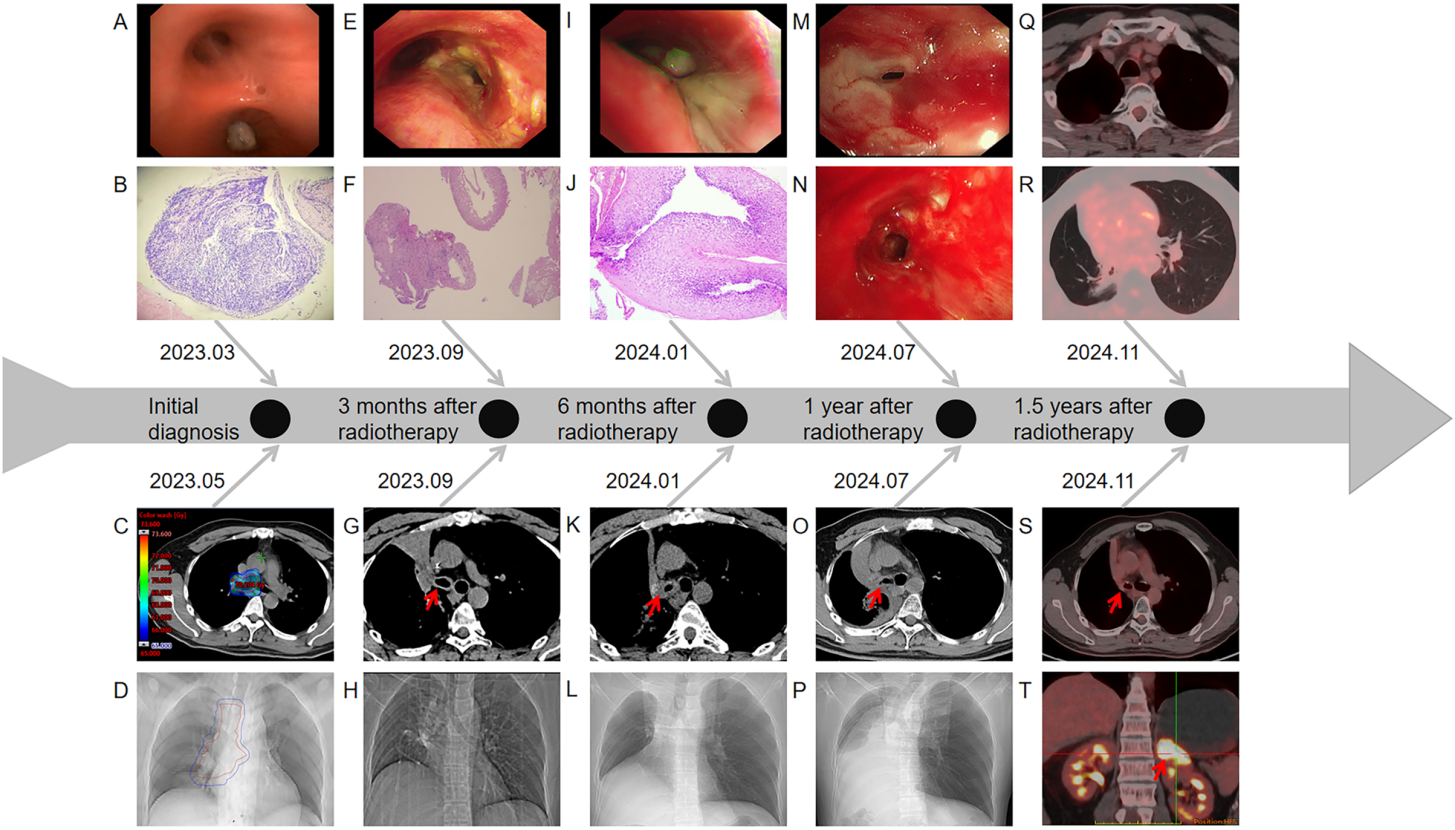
(A) Image of the tumor detected on the first bronchoscopy (March 2023). (B) Pathological results of the tumor in the right intermediate bronchus (March 2023). (C) Dose distribution in the target area at the carina. (D) Chest CT localization images before radiotherapy. (E) Image of the carina on the second bronchoscopy (September 2023). (F) Pathological results of the right middle lobe bronchus on the second bronchoscopy (September 2023). (G) Chest CT images showing changes of the carina (September 2023). (H) Chest CT localization images (September 2023). (I) Image of the carina on the third bronchoscopy (January 2024). (J) Pathological results of the right main bronchus on the third bronchoscopy (January 2024). (K) Chest CT images showed changes of the carina (January 2024). (L) Chest CT localization images (January 2024). (M) Image of the opening of the right main bronchus on the fourth bronchoscopy (July 2024). (N) Image of the opening of the right main bronchus after balloon dilatation (July 2024). (O) Chest CT images showing changes of the carina (July 2024). (P) Chest CT localization images (July 2024). (Q) Image of the mediastinal window on PET-CT (November 2024). (R) Image of the lung window on PET-CT (November 2024). (S) PET-CT imaging showing changes of the carina after balloon expansion surgery (November 2024). (T) PET-CT indicated left adrenal metastasis (November 2024).
